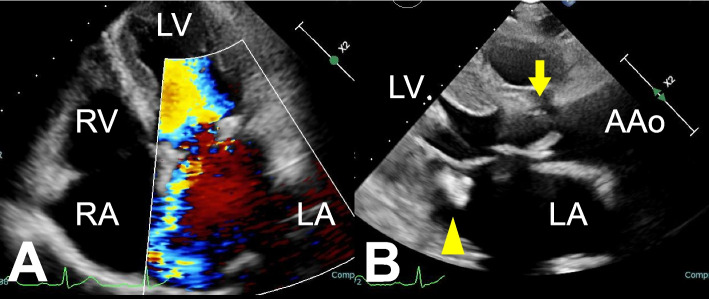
Preoperative transthoracic echocardiography. A Severe mitral regurgitation due to the posterior leaflet prolapse. B A 12-mm highly mobile mass was present on the posterior leaflet (yellow arrowhead), and the INTUITY aortic valve had thickened cusps without regurgitation (yellow arrow). AAo, ascending aorta; RA, right atrium; LA, left atrium; RV, right ventricle; LV, left ventricle
